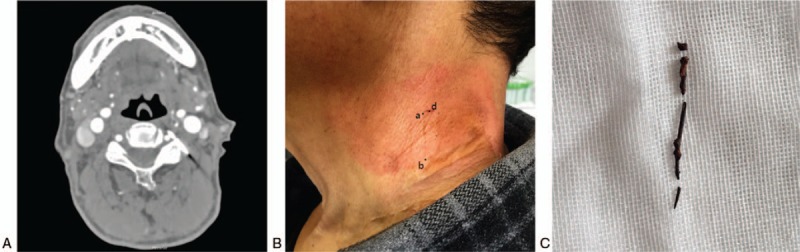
Surgical incision markers on the skin of the neck. (A) CT image of a needle-shaped foreign body near the cervical vertebral artery. (B) Postoperative image of the patient with the needle-shaped foreign body near the cervical vertebral artery (ab represents the location of the body-surface marker line; d represents the incision). (C) Extracted foreign body.
